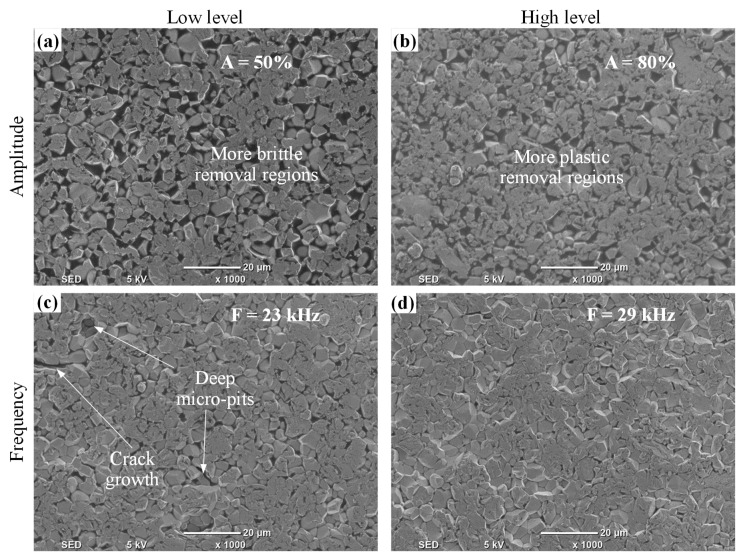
Effects of ultrasonic parameters on machined surface morphology at constant values (mid-ranges) on the remaining parameters: (a,b) effects of amplitudes and (c,d) effects of frequency.
